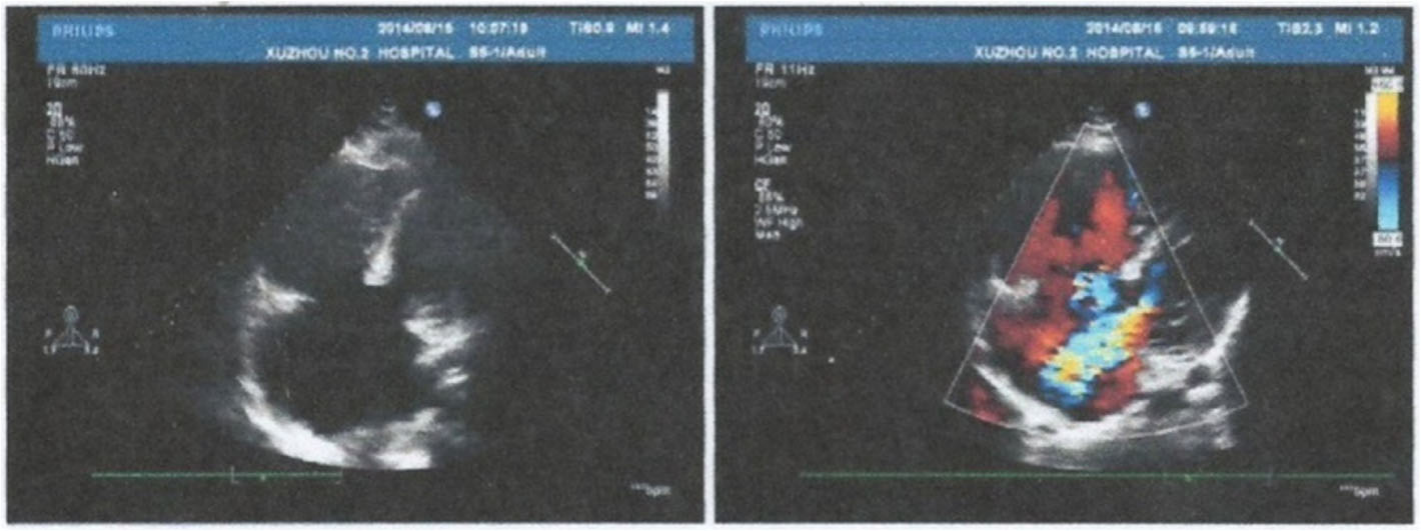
Echocardiogram of the patient’s heart, showing a single atrium. The following parameters were described: pulmonary artery, 27 mm; right ventricular diameter, 39 mm; left ventricular end diastolic diameter (LVEDD), 43 mm; left ventricular ejection fraction (LVEF), 51%; E/A, 1.01/0.69; pulmonary pressure, 16 mmHg. The echocardiogram showed evidence of congenital heart disease (CHD), including no visible nub of the interatrial septum on any section, a single atrium (SA), pulmonary artery enlargement, right ventricular hypertrophy, mitral and tricuspid valves located at the same level, poor valve development, mitral valve moderate regurgitation, tricuspid moderate regurgitation, and disappearance of the endocardial cushion decussation
